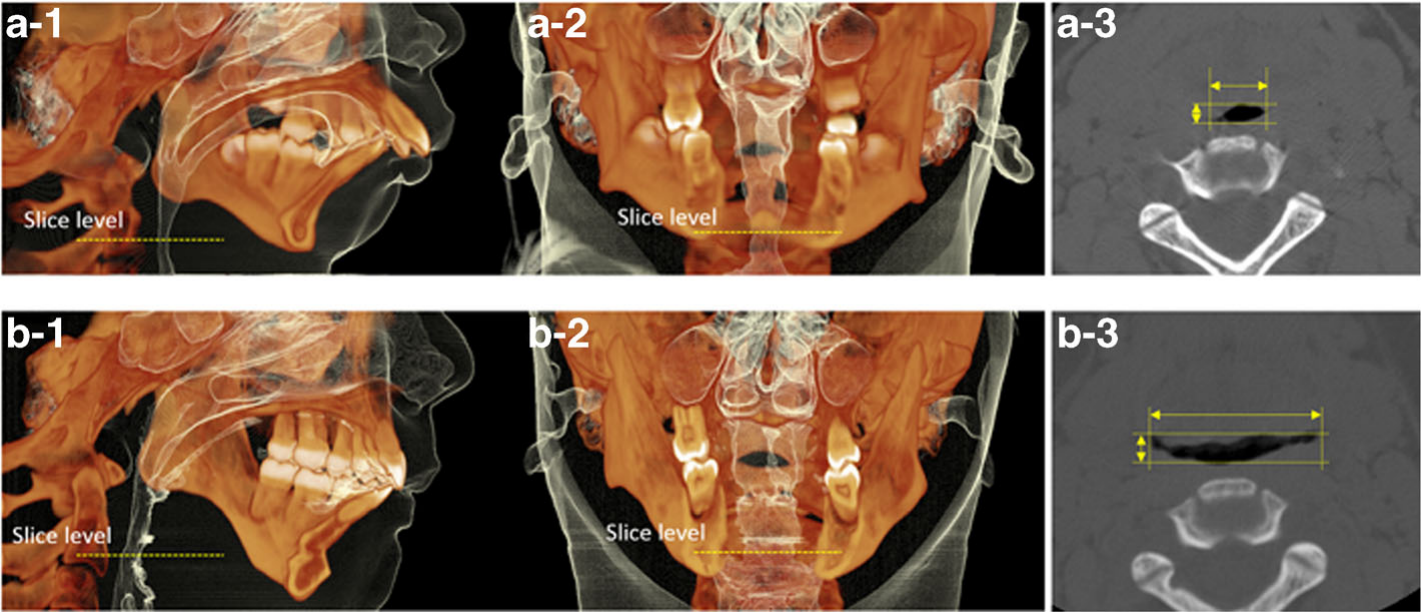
Volume rendering computed tomography images of the upper airway. Lateral view (a-1), frontal view (a-2), and transverse and anteroposterior airway diameters in the retroglossal region (a-3) at pre-treatment; lateral view (b-1), and frontal view (b-2), transverse and anteroposterior airway diameters in the retroglossal region (b-3) at post-treatment
